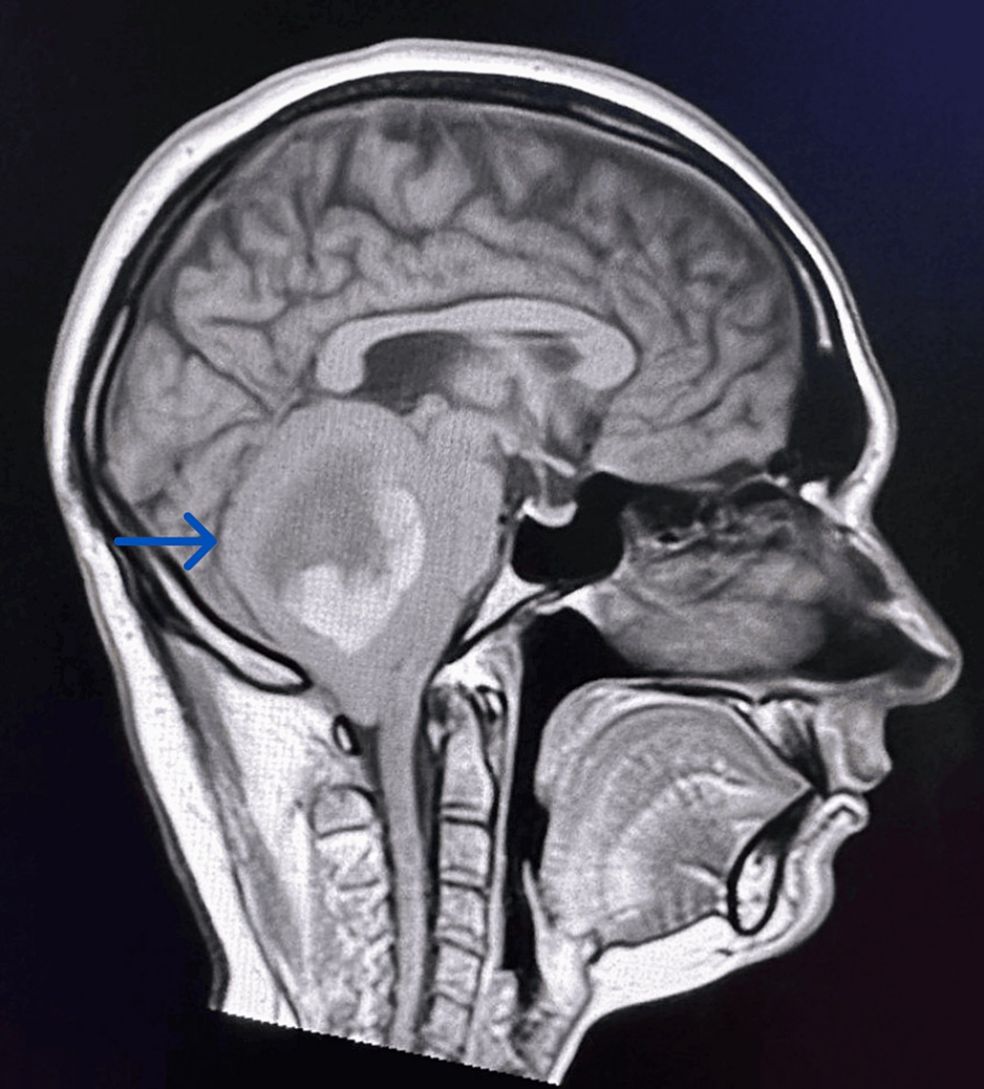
Initial diagnostic magnetic resonance imaging head scan of the patient with axial T1 contrast views demonstrating a cerebral lesion.
